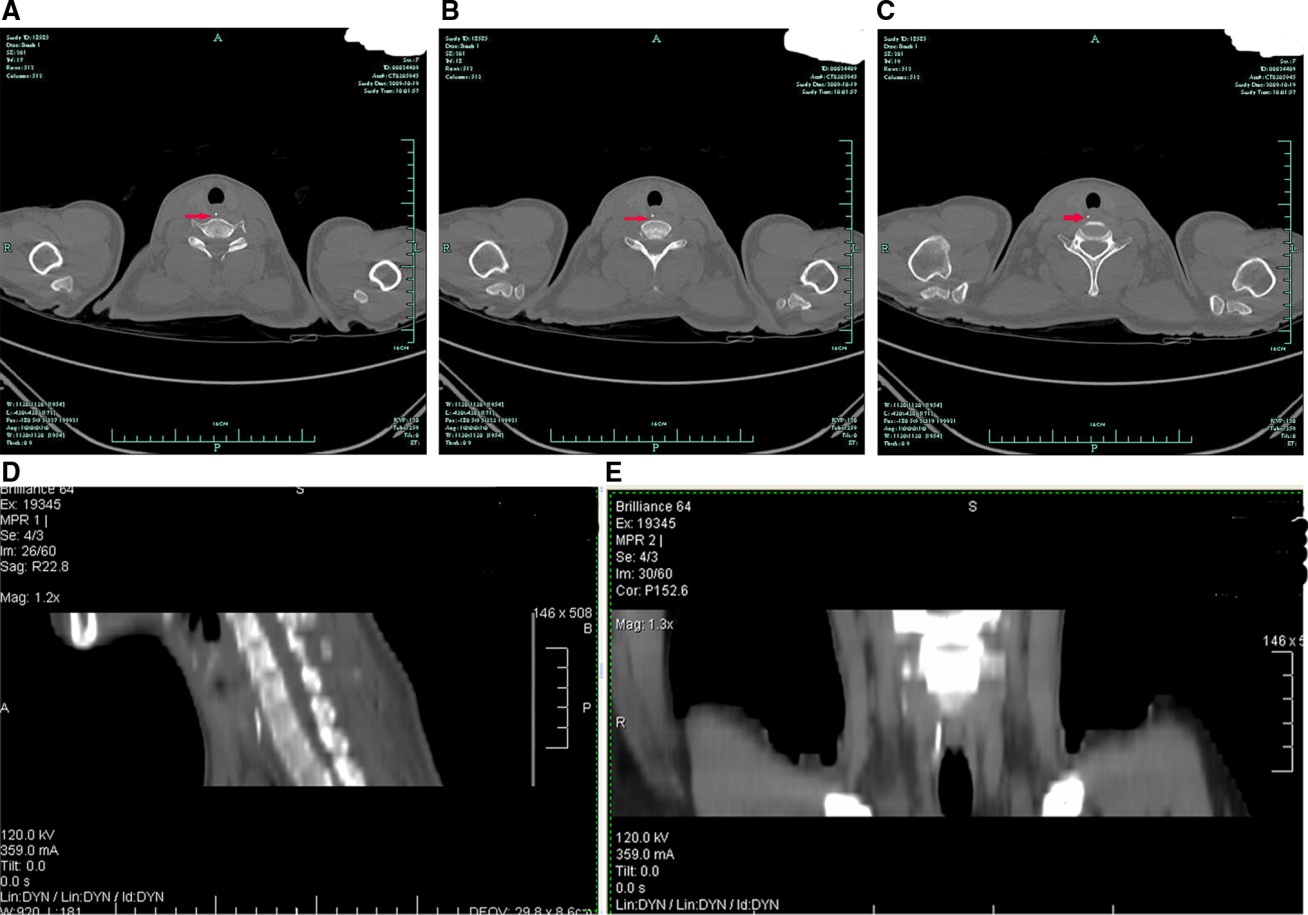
The day after ingestion. A computed tomography (CT) scan revealed a linear hyperdensity located next to the esophageal lumen, indicating that the FB had penetrated the esophageal wall. a–c Plain transverse images revealing the FB (*arrow*) in the cervical esophagus, d sagittal MPR revealing that the FB had moved downward and was now in front of C7, with its long axis paralleling the longitudinal esophageal axis, e coronal MPR revealing that the FB had moved into the right paraesophageal adipose space and that its long axis now paralleled the esophageal longitudinal axis
